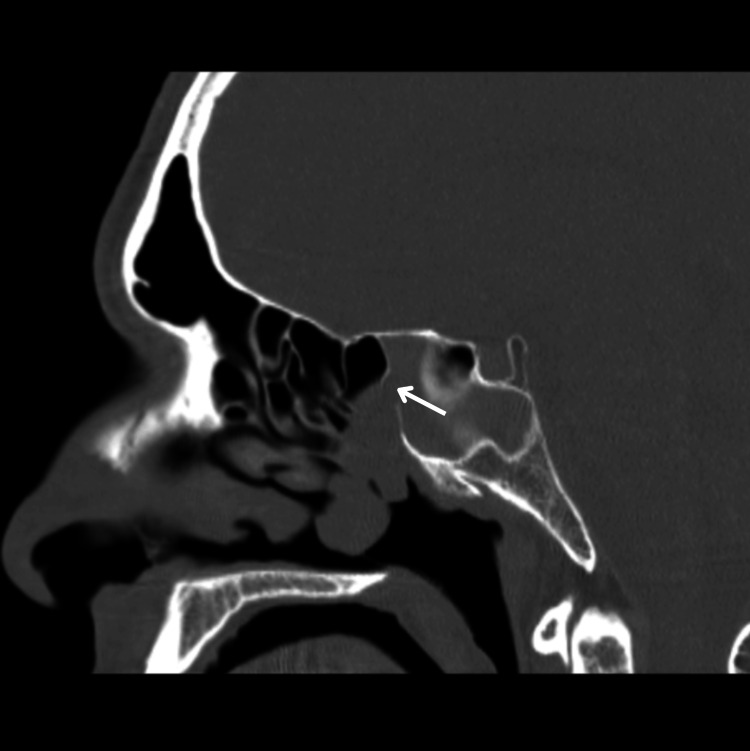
CT head (sagittal view) showing an obliterated right sphenoid sinus ostium (white arrow).
